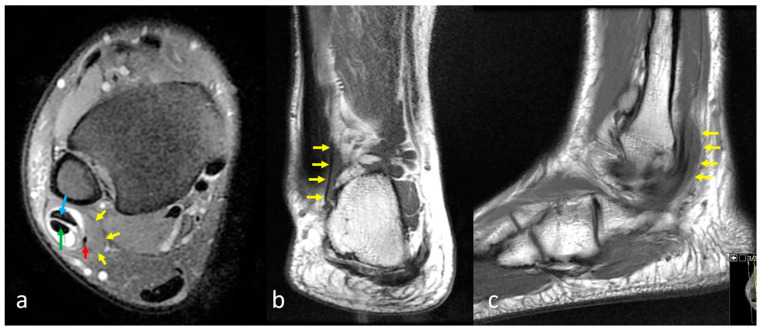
MRI appearance of the peroneus quartus (PQ) muscle in the lateral compartment of the leg. (a) Axial fat-suppressed proton density–weighted fast spin echo (FSE) image showing the peroneus brevis (blue arrow), peroneus longus (green arrow), and the accessory peroneus quartus muscle (red arrow). The PQ lies posterior to the fibula and lateral to the other peroneal tendons. Yellow arrows indicate the muscle belly of the PQ. (b) Coronal T1-weighted FSE image demonstrating the longitudinal course of the PQ muscle (yellow arrows) descending toward the calcaneus. (c) Sagittal T1-weighted fast spin echo (FSE) image demonstrating the peroneus quartus (PQ) muscle extending distally toward its insertion on the retrotrochlear eminence of the calcaneus (yellow arrows).
